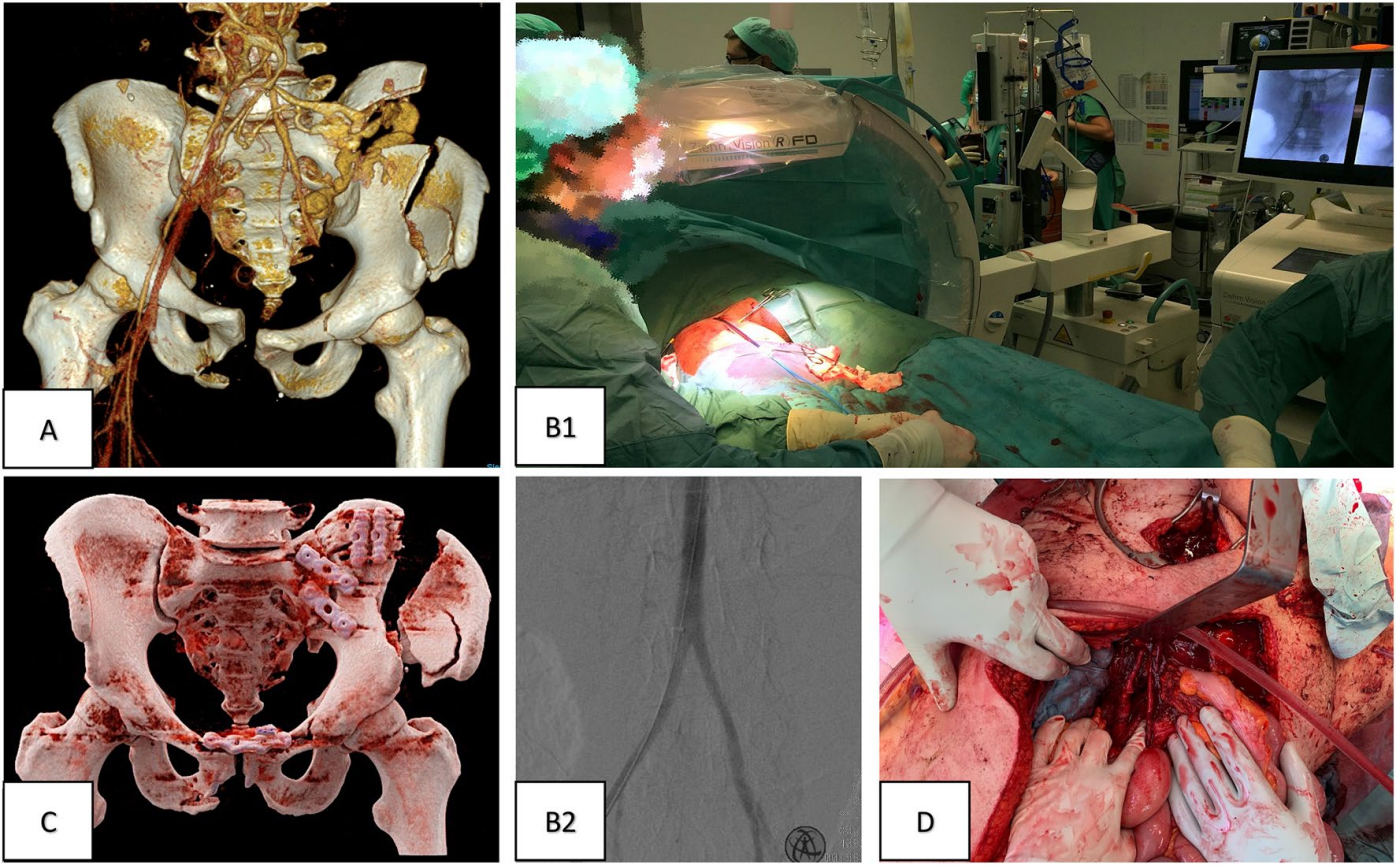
Patient 3 initial surgical strategy and control of bleeding. A MS-3D-CT scan with contrast of the obtained injury (AO classification: 61-C3.1 b, d, h, j—right iliosacral disruption—left multifragmentary iliac wing fracture; complete traumatic hemipelvectomy), occlusion of the external iliac artery, bleeding of the internal iliac artery. B Early bleeding control via clamping and packing in the resuscitation room after the application of REBOA before narcosis to prevent CPR (B1) and blocking on level III (B2). C Postoperative MS-3D-CT scan after vascular reconstruction, dermatofasciotomy of the left leg, and plate osteosynthesis of the pelvis. D Vascular reconstruction of the external iliac artery using a venous graft
